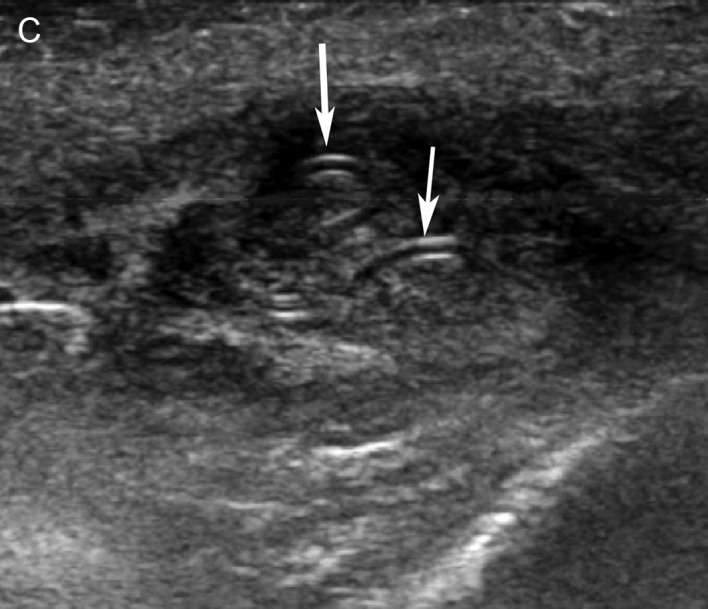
A new ultrasound scan performed immediately after the new MRI. (A) Conventional images perpendicular to the skin surface show a poorly defined, heterogeneous, hypoechoic deep hypodermal mass containing rectilinear double echogenic structures several millimeters long (arrows) or very short, almost point-like (arrowheads). (B) Color Doppler examination shows clear hypervascularization. (C) A nearly ‘horizontal’ slice, close to a plane parallel to the skin, taken after more than 10 minutes of examination, shows that the double echogenic structure has curved portions (arrows). It is at this stage that spontaneous movements of the structure were observed (see Figure 7).
